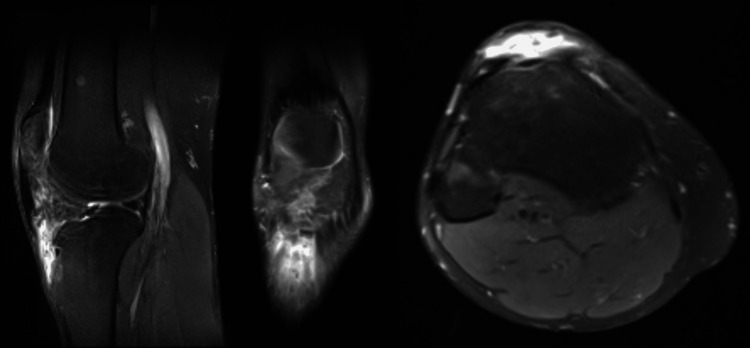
MRI of the injured knee (left to right: sagittal, coronal, transversal view)
